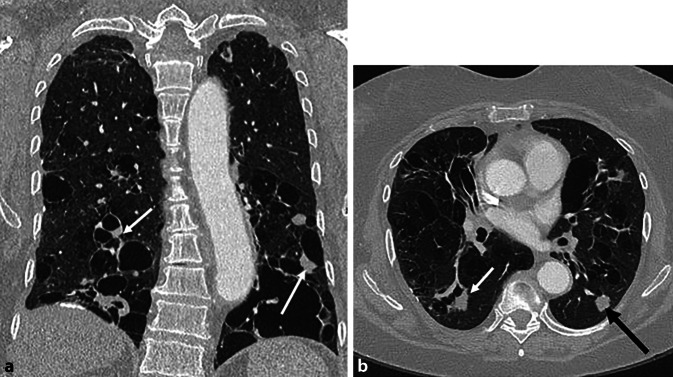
a Koronare Rekonstruktion. b Axiale CT-Thorax auf Höhe der Mittel- und Unterlappen. Äußerst seltene Manifestationsform der pulmonal-parenchymalen Amyloidose in Form einer zystischen Lungenerkrankung bei einem Patienten mit Leichtkettenamyloidose. Es sind multiple Lungenzysten mit basaler Verteilungsdominanz erkennbar sowie pathognomonische perizystische Noduli (*weiße*
